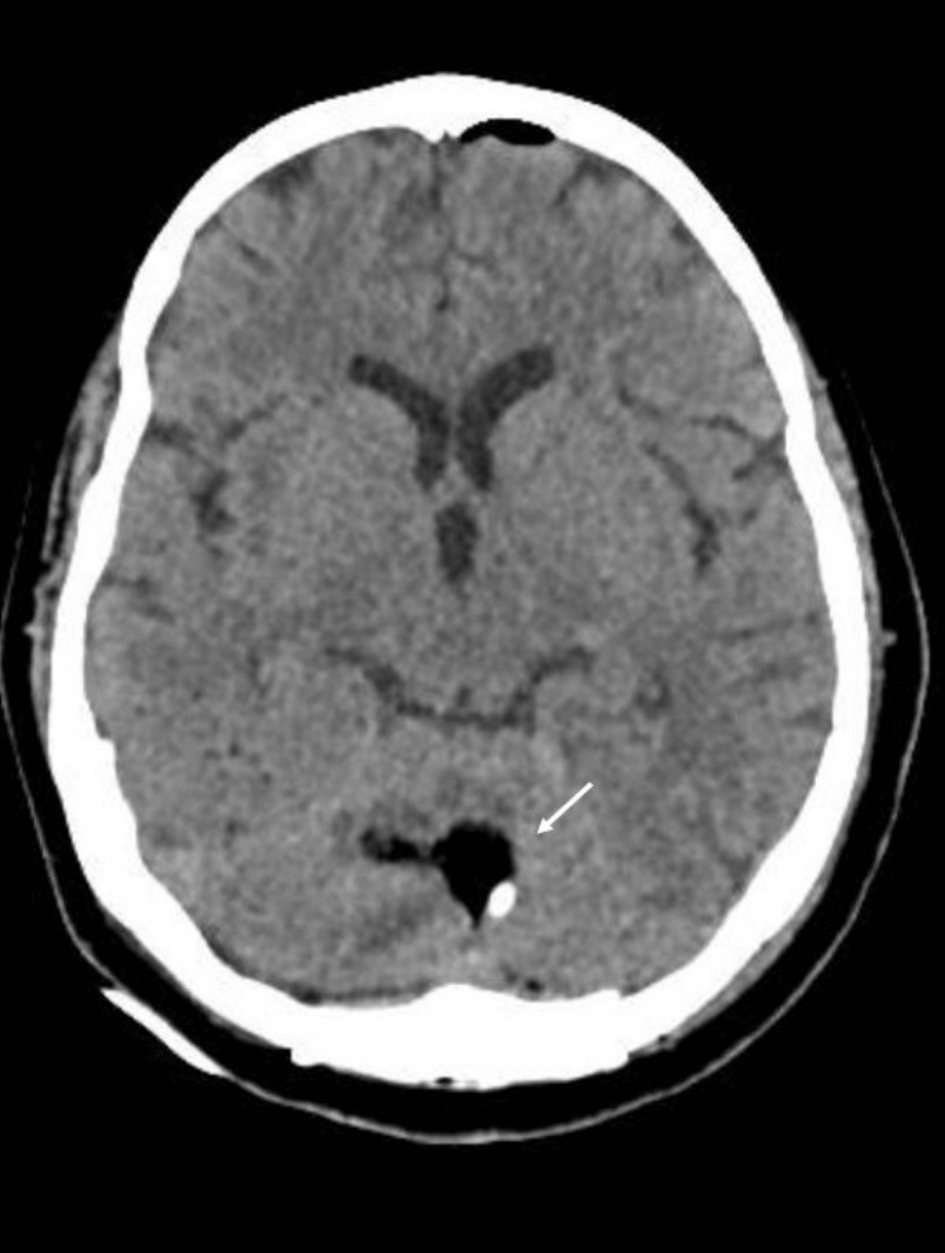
Postoperative axial non-contrasted CT showing the operative site (white arrow). CT: computed tomography
